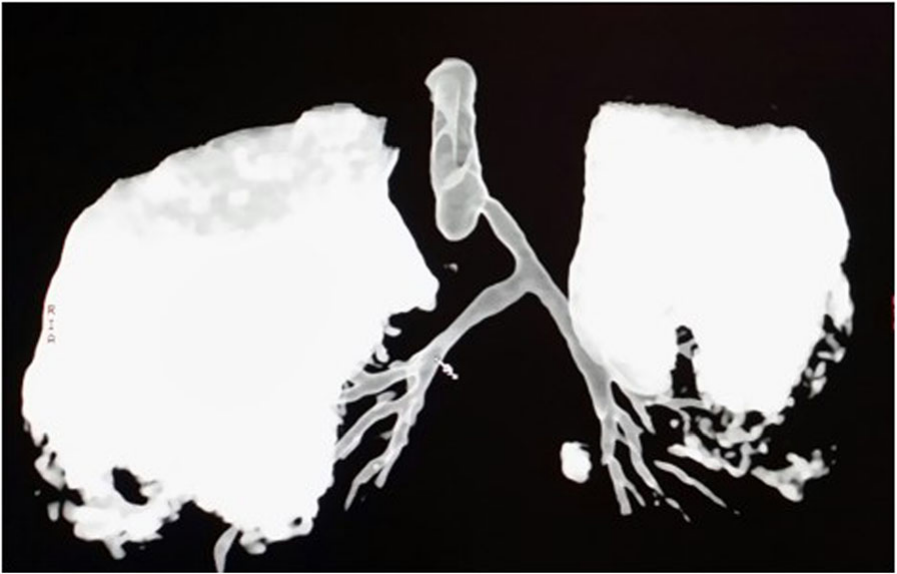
Three-dimensional reconstruction CT of the tracheobronchial tree
